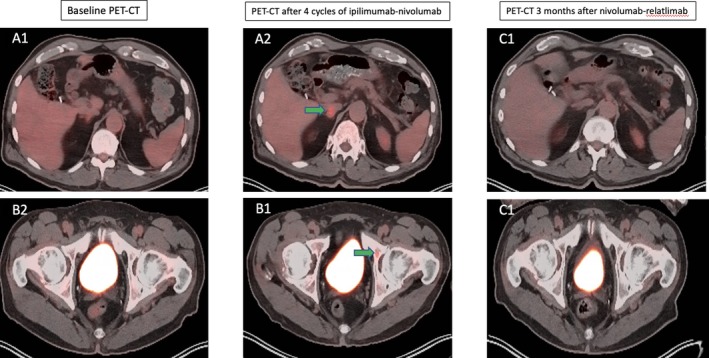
PET‐CT images demonstrating disease status before and after ipilimumab‐nivolumab and nivolumab‐relatlimab regimens. (A2 and B2) FDG‐PET showing new hypermetabolic right adrenal (A2) and left acetabular lesions (B2) in comparison to baseline images (A1 and B1) showing no such lesions, thus consistent with distant disease progression after 4 cycles of ipilimumab plus nivolumab. (C1 and C2) Interval resolution of right adrenal and left acetabulum hypermetabolic activity 3 months following nivolumab plus relatlimab therapy.
